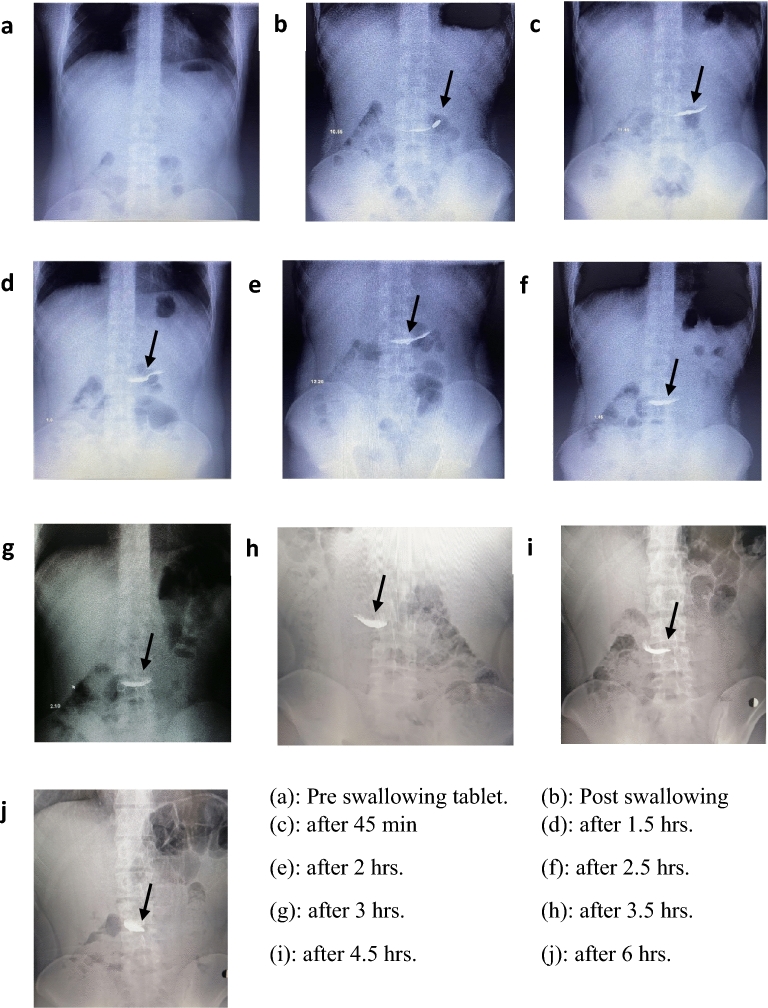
Radiographic images of F6 at different times after oral administration.
